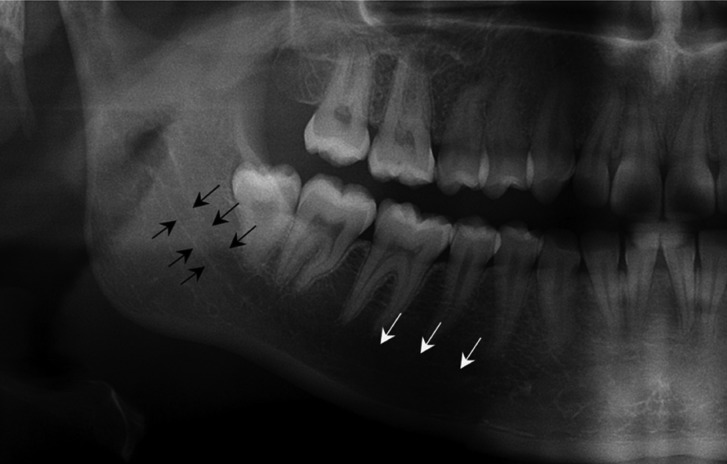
Cropped panoramic radiograph showing mandibular canal cortication (black arrows) and submandibular fossa region (white arrows).
